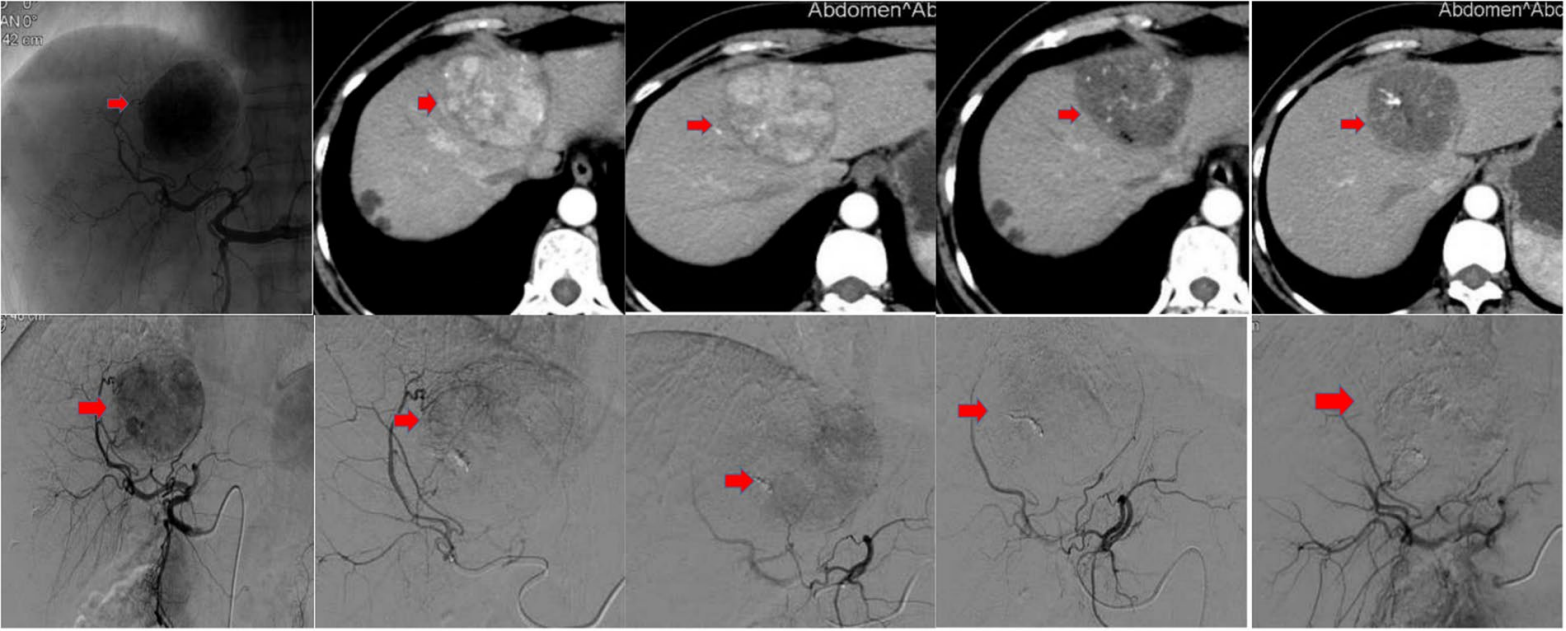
CT images of a 53-year-old female patient diagnosed with HCC. The Top left CT image shows a large tumor in the left liver lobe (May 18, 2017). The top middle images show residual enhancement of the tumor in the left liver lobe (June 20, 2017). The bottom CT images show TACE with doxorubicin 50 mg and CalliSpheres of 300–500 µm (June 22, 2017). The patient reported fever and abdominal pain after the TACE procedure, but no biloma was detected (top right, June 26, 2017).
